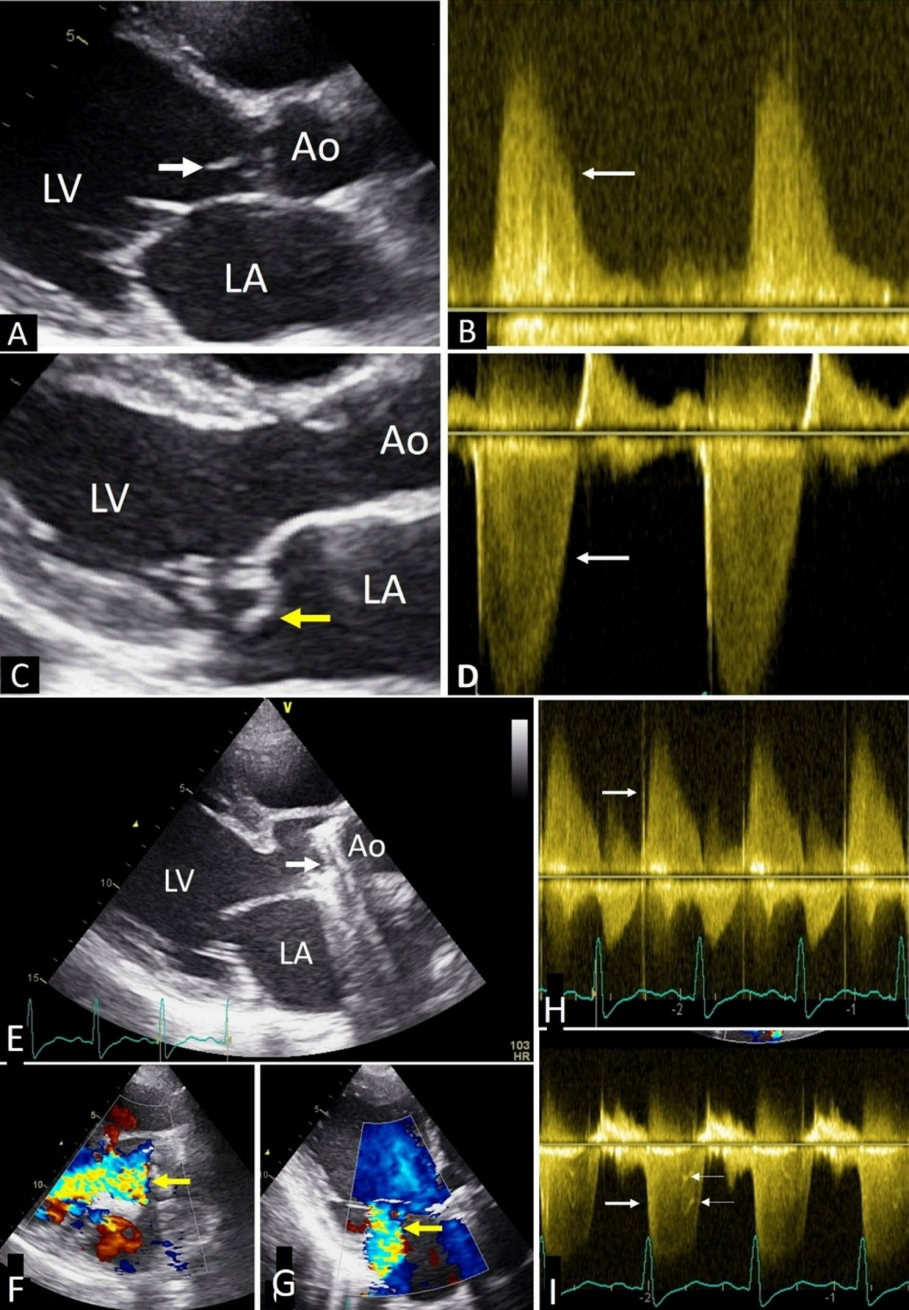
Tiger stripes are absent in cases with oscillating structures in flow pathways. (A) Vegetation (white arrow) in aortic valve seen in parasternal long axis view of trans thoracic echo (see video 4). (B) CW Doppler spectrum of acute aortic regurgitation in same patient in apical 5 chamber view (arrow) without any stripe. (C) Ruptured chordae prolapsing to left atrium in systole (yellow arrow) seen in parasternal long axis view (see video 5), (D) CW Doppler spectrum of the same patient in apical 4 chamber view have no stripe seen (arrow). (E-I). A case of aortic mechanical prosthetic valve dehiscence, (E), arrow shows the prosthetic valve in a 2-dimensional transthoracic echocardiographic image in parasternal long axis view, (F) and (G), arrows show the aortic regurgitant and mitral regurgitant jets respectively in long axis view. (H), CW Doppler spectrum of acute aortic regurgitation spectrum does not show tiger stripe (arrow). (I), tiger stripes are present in the CW Doppler spectrum of mitral regurgitant jet (small arrows). LV-Left ventricle, LA-Left atrium, Ao-Aorta
